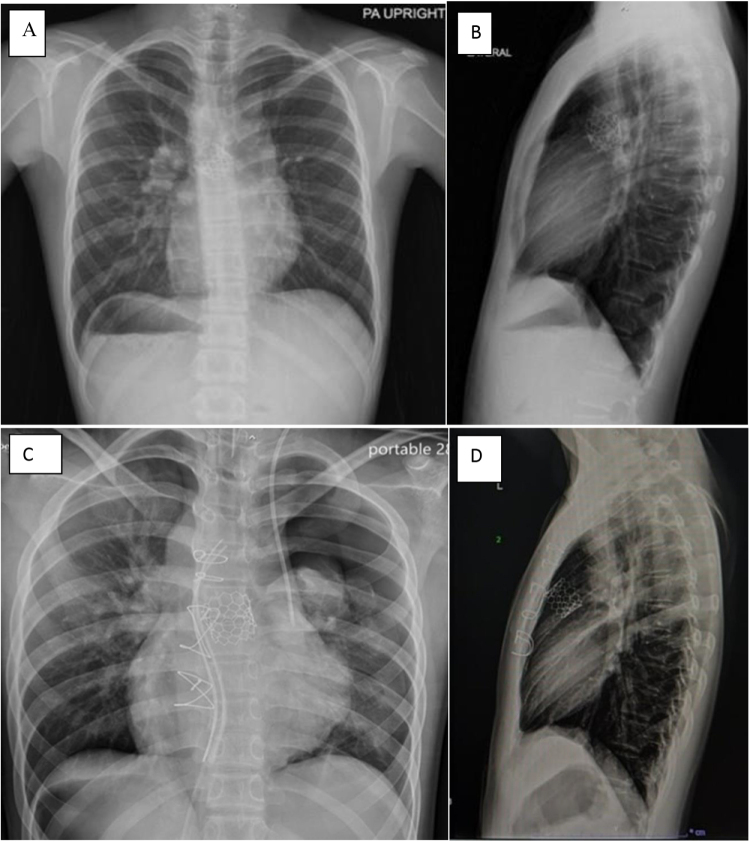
Chest X-ray PA (A) and lateral views (B) post placement of the MyVal valve percutaneously. Chest X-ray PA (C) and lateral views (D) post repositioning surgically.
